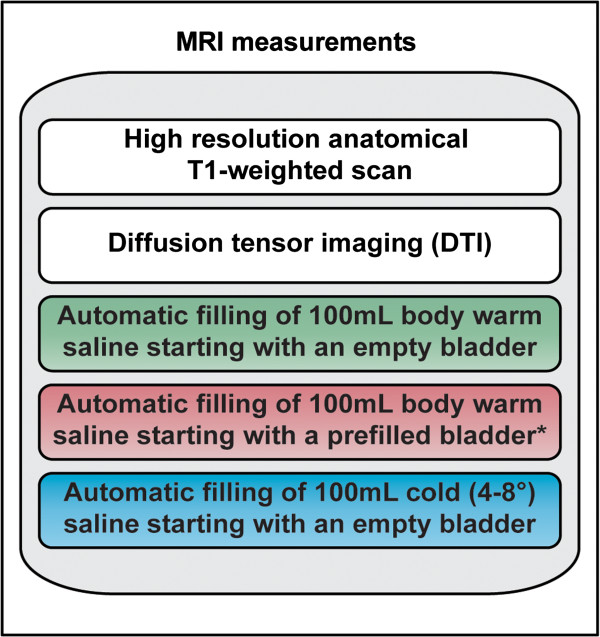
Sequences of magnetic resonance imaging (MRI) measurements. *Bladder will be filled with body warm saline until a persistent desire to void is present.
